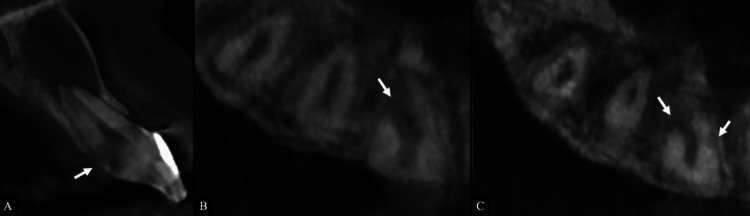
CBCT images of tooth #11 demonstrating ECR. (A) Sagittal view showing a palatal ECR defect extending to the middle third of the root (white arrow). (B, C) Axial views demonstrating circumferential spread ranging from >180° to ≤270°, with evidence of pulpal communication (white arrows). CBCT: Cone beam computed tomography
